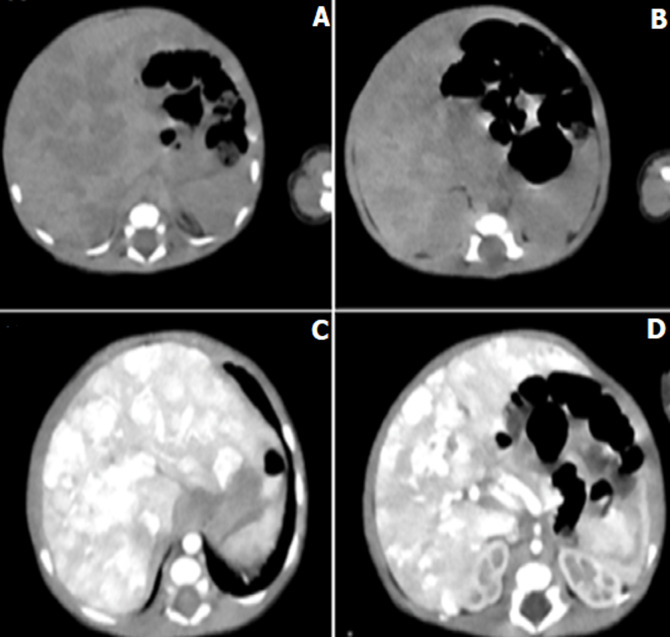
A,B) abdominal CT scan without injection; C,D) after injection showing multiple hepatic angiomas
